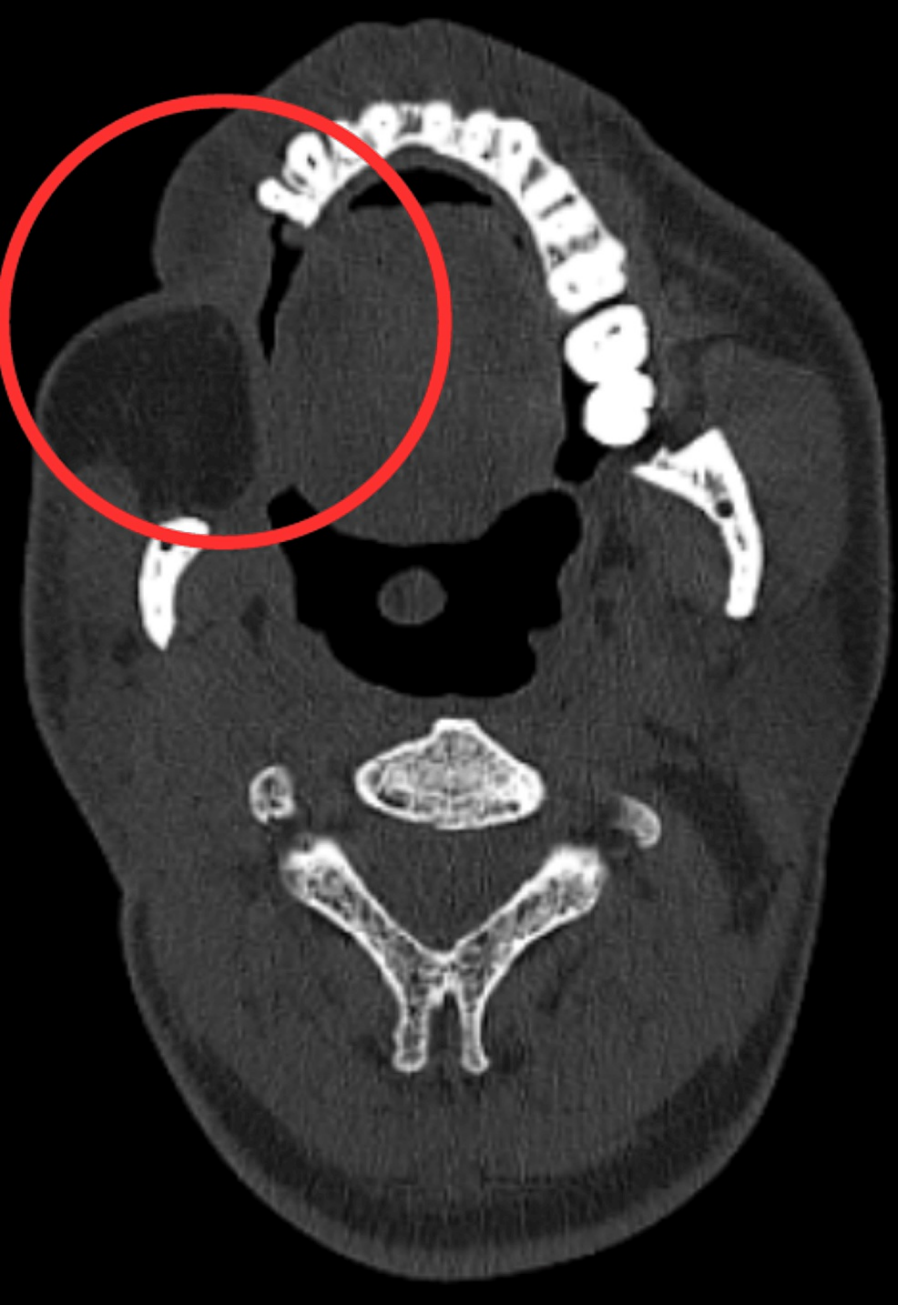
CECT of the buccal cavity CECT, contrast-enhanced computed tomography
